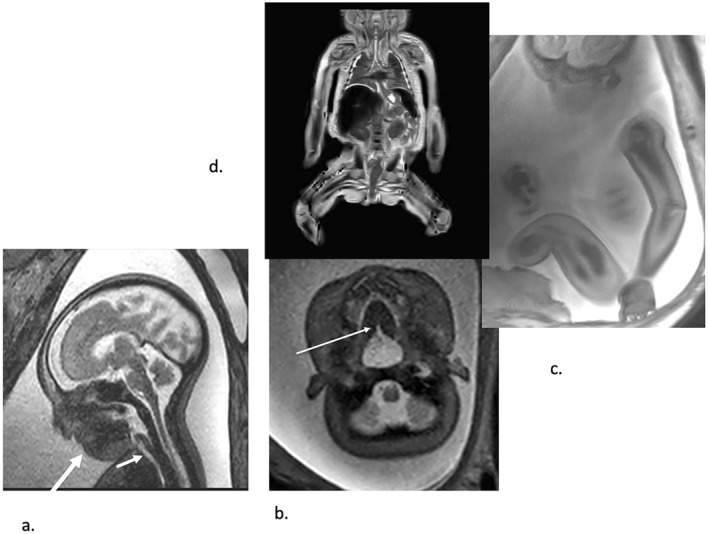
Fetus at 36 weeks' gestation with biopsy‐proven Type 2 muscle fiber deficiency. (a) Sagittal T2‐weighted echoplanar image showing abnormal fetal profile due to micrognathia (long arrow) and there is polyhydramnios but the jaw index was greater than the 5th percentile at 33. There is also no evidence of glossoptosis or oropharyngeal or tracheal (short arrow) obstruction. (b) A very small posterior palate cleft is seen on this axial T2‐weighted echoplanar image (arrow). (c) Sagittal T2‐weighted echoplanar image demonstrating gross muscular underdevelopment in the arm and thigh, confirmed on coronal T2‐weighted fast spin echo post‐mortem MRI image (d). Polyhydramnios was due in this case to neuromuscular dysfunction rather than micrognathia‐related airway obstruction.
